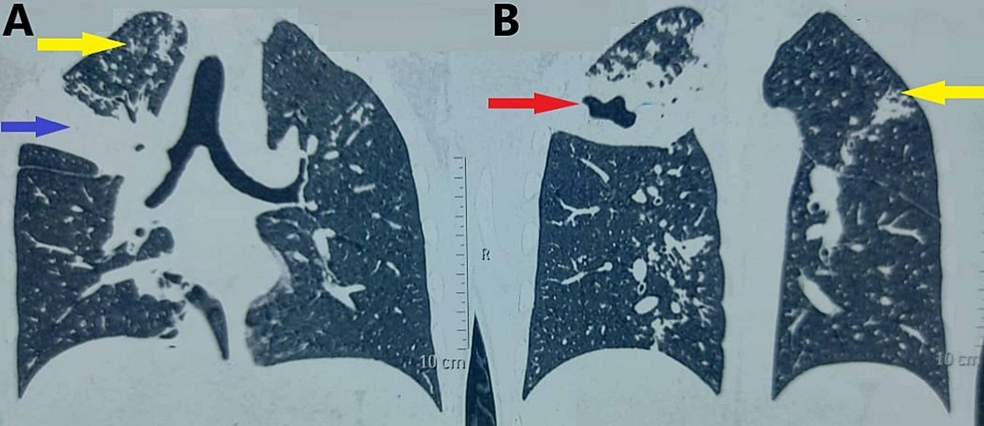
Coronal sections (A and B) of HRCT scan of the chest showing consolidation (blue arrow) and cavity (red arrow) in the right upper lobe along with bilateral branching centrilobular nodules (yellow arrows). HRCT: High-resolution computed tomography
